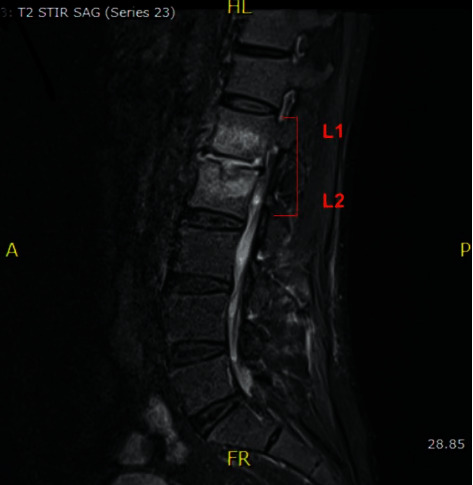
MRI of the spine with paraspinal soft tissue swelling and enhancement at the level of L1 and L2 as well as ventral epidural enhancement without rim-enhancing intraspinal or paraspinal fluid collections.
